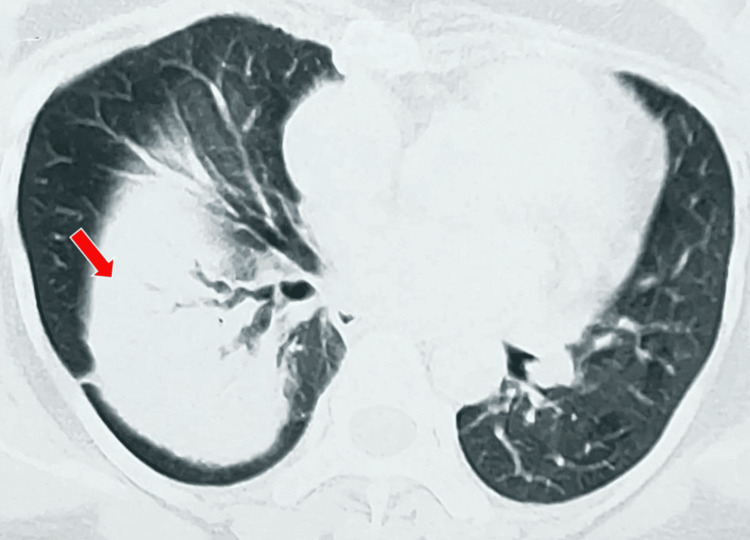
A CT scan of the chest showing soft tissue density (red arrow) in the right lower bronchus.
